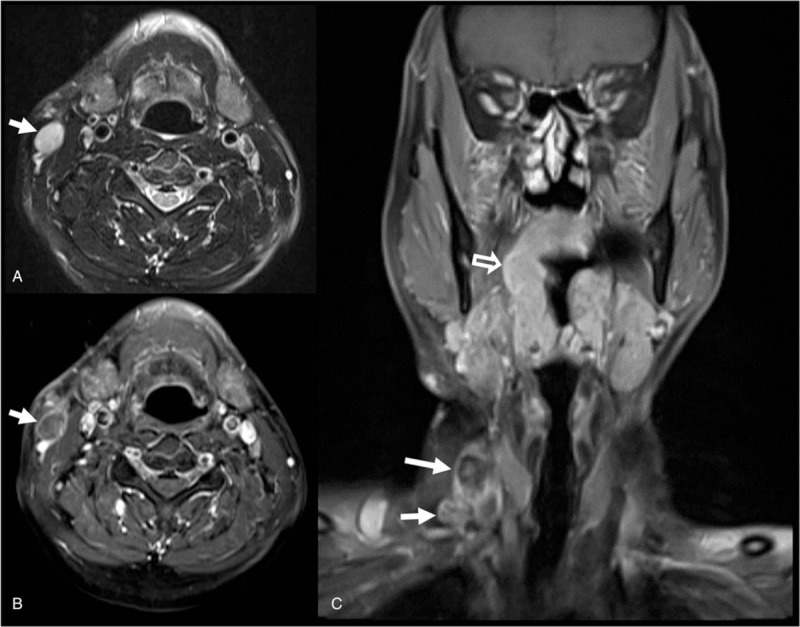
Follow-up head and neck* magnetic resonance images. (A) Axial T2-weighted image and (B) axial contrast-enhanced T1-weighted image. Both show a recurrent cystic lesion (arrow) measuring 2.19 × 1.82 × 1.35 cm between the platysma and sternocleidomastoid muscle at the hyoid bone level. (C) Coronal contrast-enhanced T1-weighted image with fat suppression shows new cystic lesions at Level IV (arrows) and Level I (not shown). Prominent right palatine tonsil is also noted (outline arrow).
